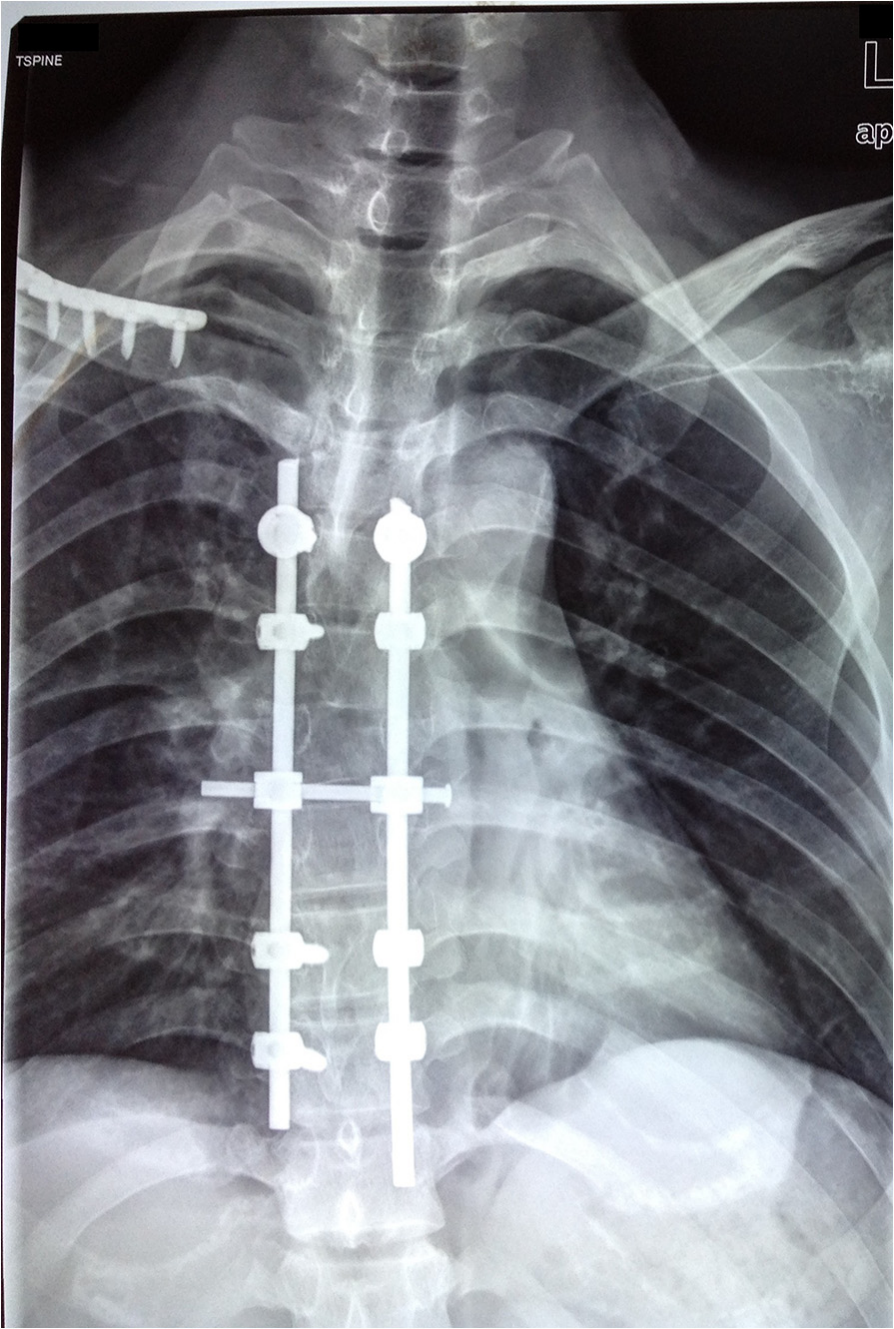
Anteroposterior thoracic spine plain X-ray showed realignment of the fractured-dislocated spine.
